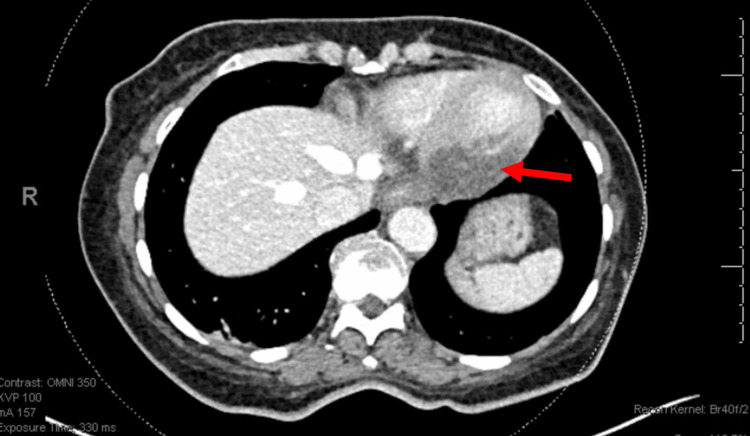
CT scan demonstrating hypoattenuation of the posteroinferior aspect of the left ventricular wall, concerning for acute myocardial infarction.
